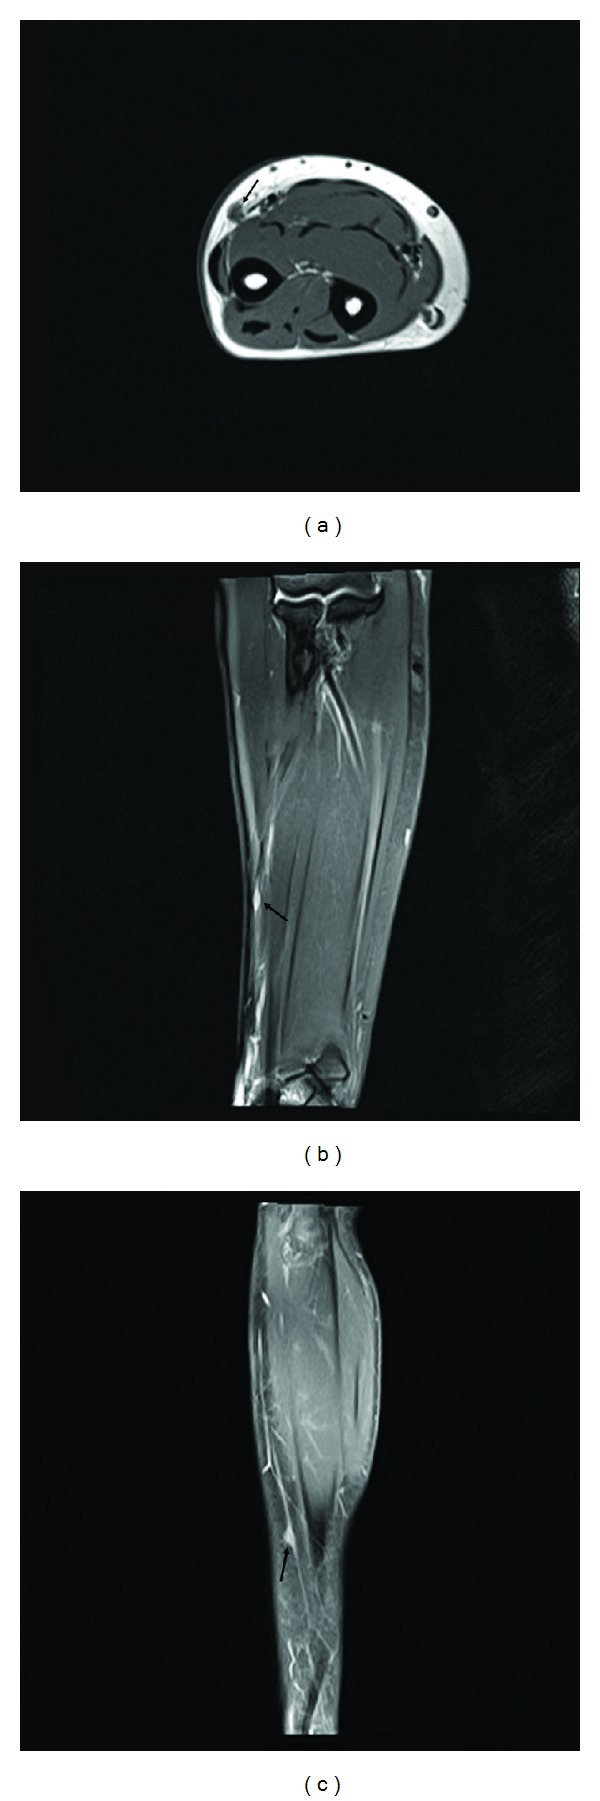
(a) MRI axial T1-weighted image showing an intermediate intensity mass on the subcutaneous layer of the volar-radial aspect of the forearm (arrow). (b) MRI coronal T2-weighted image showing a well-defined high signal mass that is especially prominent in the small vessel (arrow). (c) MRI sagittal image with contrast displaying a homogenous strong enhancement (arrow).
